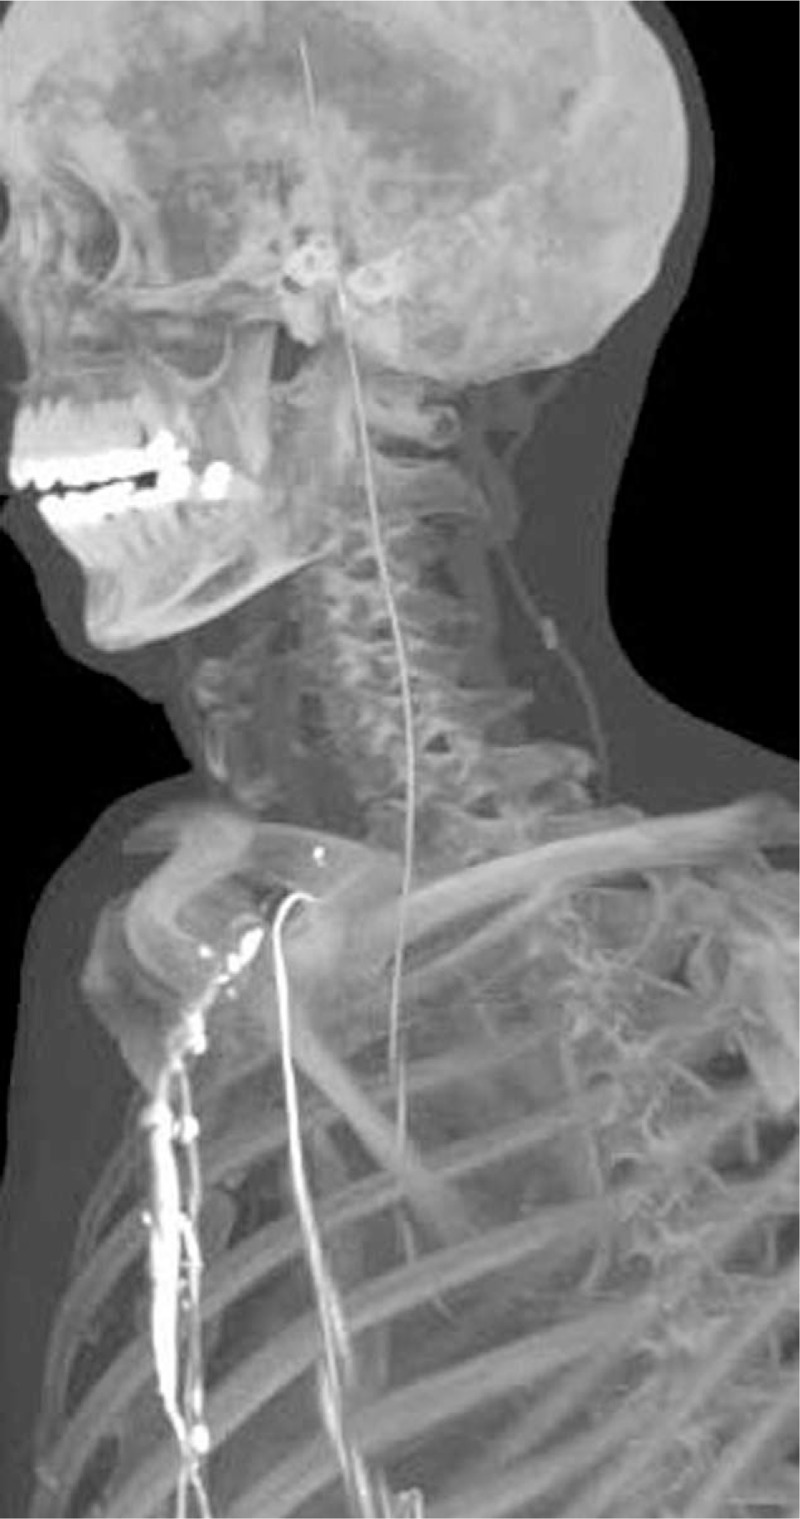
3D reconstruction of computed tomography showing the position of the guide wire in the cerebrum.
